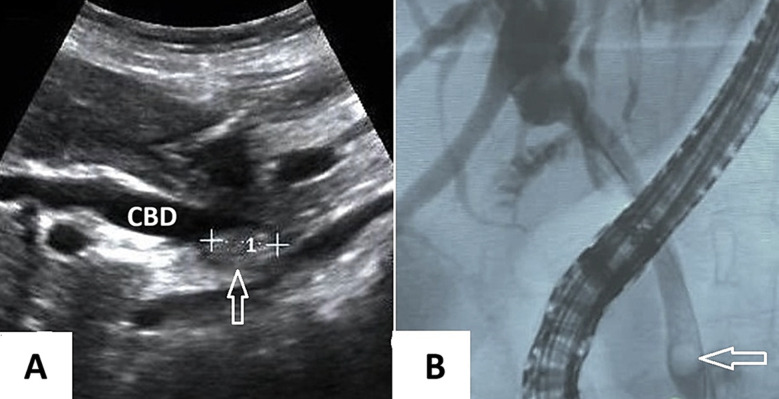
A) A Transabdominal Ultrasonography image showing dilatation of the common bile duct (CBD) with a large stone (arrow) impacted near the distal part of it. B) A cholangiography image showing dilatation of the CBD and biliary ducts with a filling defect (arrow) at the distal end, which proved to be a CBD stone.
